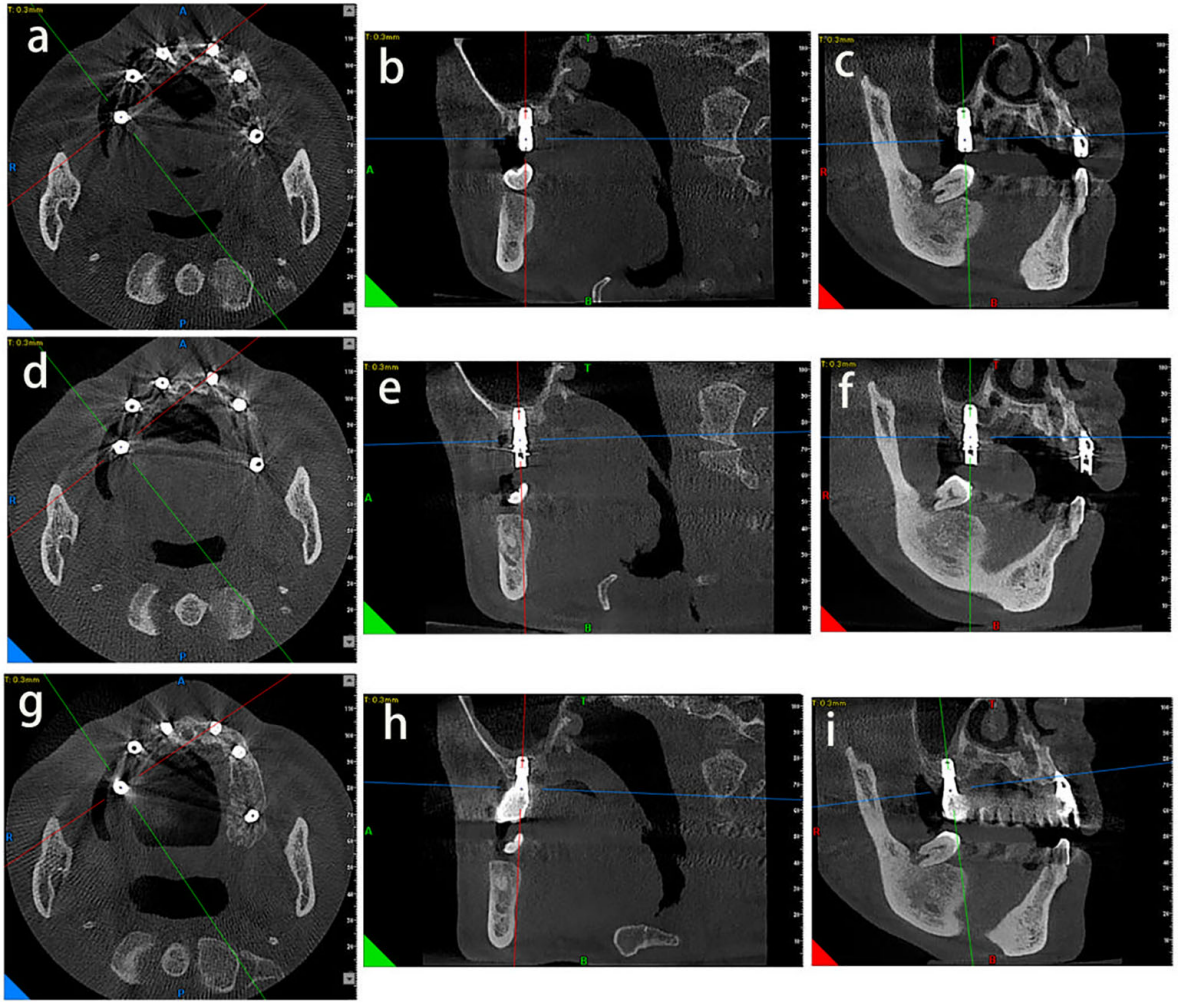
The measurement results at different time points after immediate implant placement. (a-c) Measurement results were measured after immediate implant placement at axial, sagittal and coronal CBCT scans immediately. (d-f) Measurement results were measured at 3months after immediate implant placement at axial, sagittal and coronal CBCT scans. (g-i) Measurement results were measured at 6 months after immediate implant placement at axial, sagittal and coronal CBCT scans. The tooth bit is 16 in this patient.
